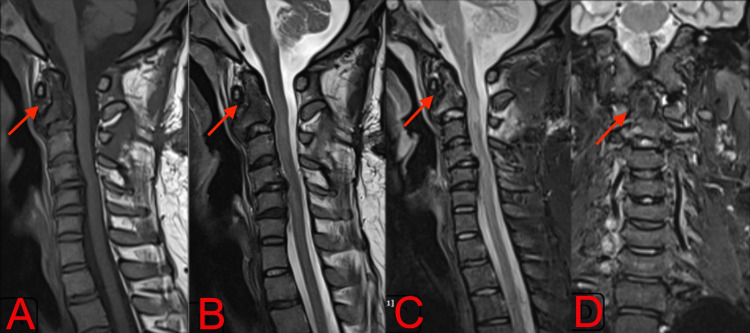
At the two-year follow-up, T1 (A), T2 (B), and STIR (C, D) MRI images showed sclerosis of C2 vertebra with clearance of the spinal canal. MRI: Magnetic Resonance Imaging, STIR: short tau inversion recovery
